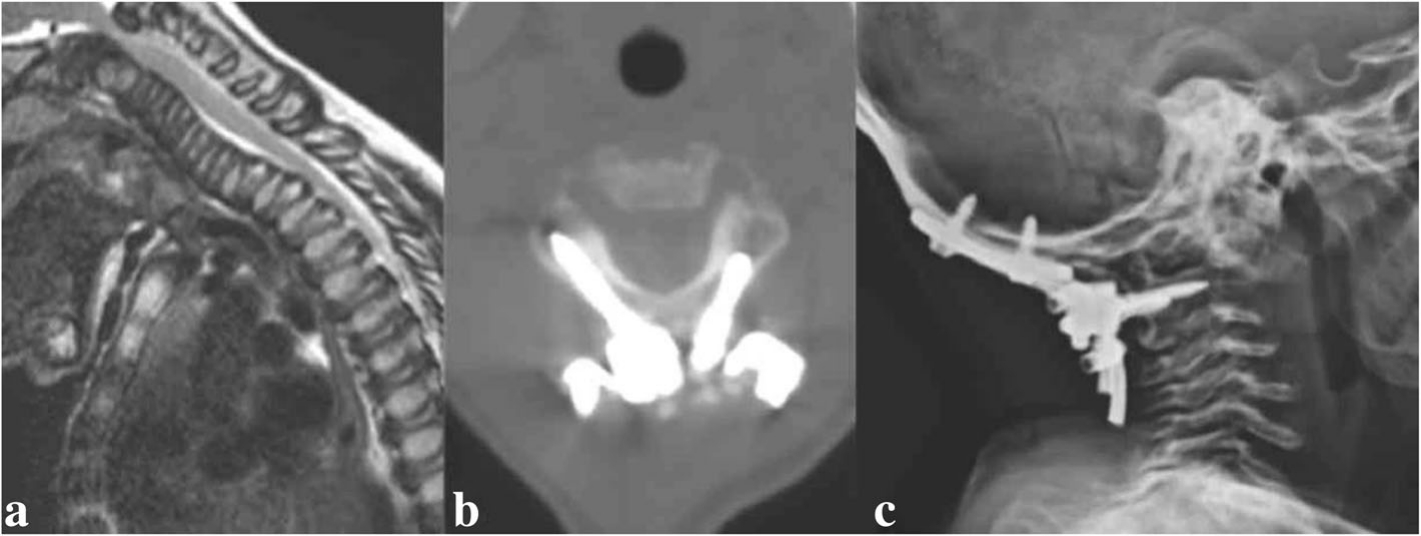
a MRI showing severe cord compression during head flexion in MPS IVA, b laminar screws and c postoperative plain x-rays at 2 years follow-up
